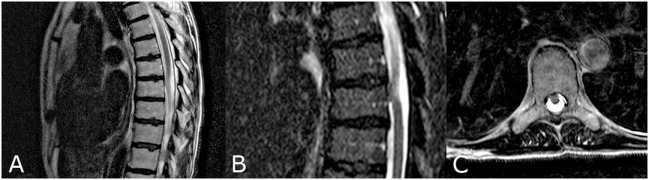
MRI of second patient disclosing a herniated spinal cord at T8 at sagittal T2 weighted image (A), at a STIR weighted image (B), and an axial T2 weighted Image (C).
